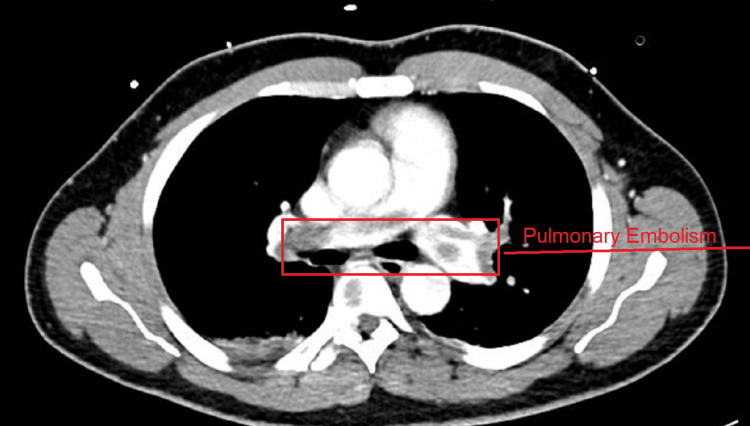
CT of the thorax with contrast showing massive pulmonary embolism within the left and right pulmonary arteries.
